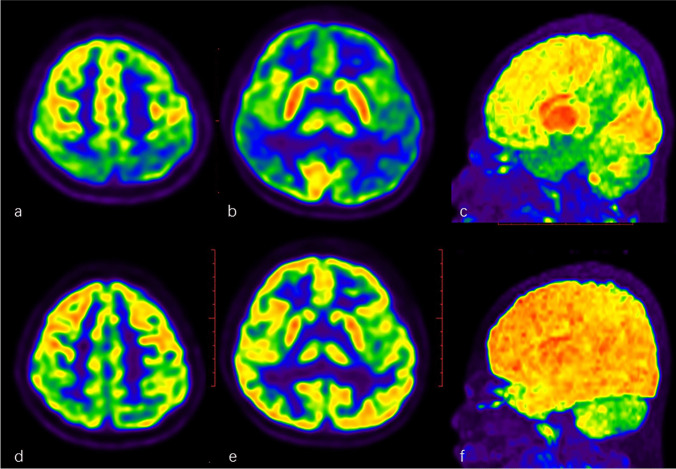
A 7-year-old boy with a 6-year history of seizures and developmental retardation was finally diagnosed with seronegative AE. Brain ^18^F-FDG PET/CT showed large-scale hypometabolism in bilateral parietal, temporal, and frontal lobes with focal hypermetabolism in basal ganglia (a, b, c). 2.5 months later, the second brain ^18^F-FDG PET/CT scanning indicated the significant recovery of the abnormal metabolism (d, e, f) after immunotherapy, consistent with the improvement of clinical symptoms. c, f MIP map of FDG PET
